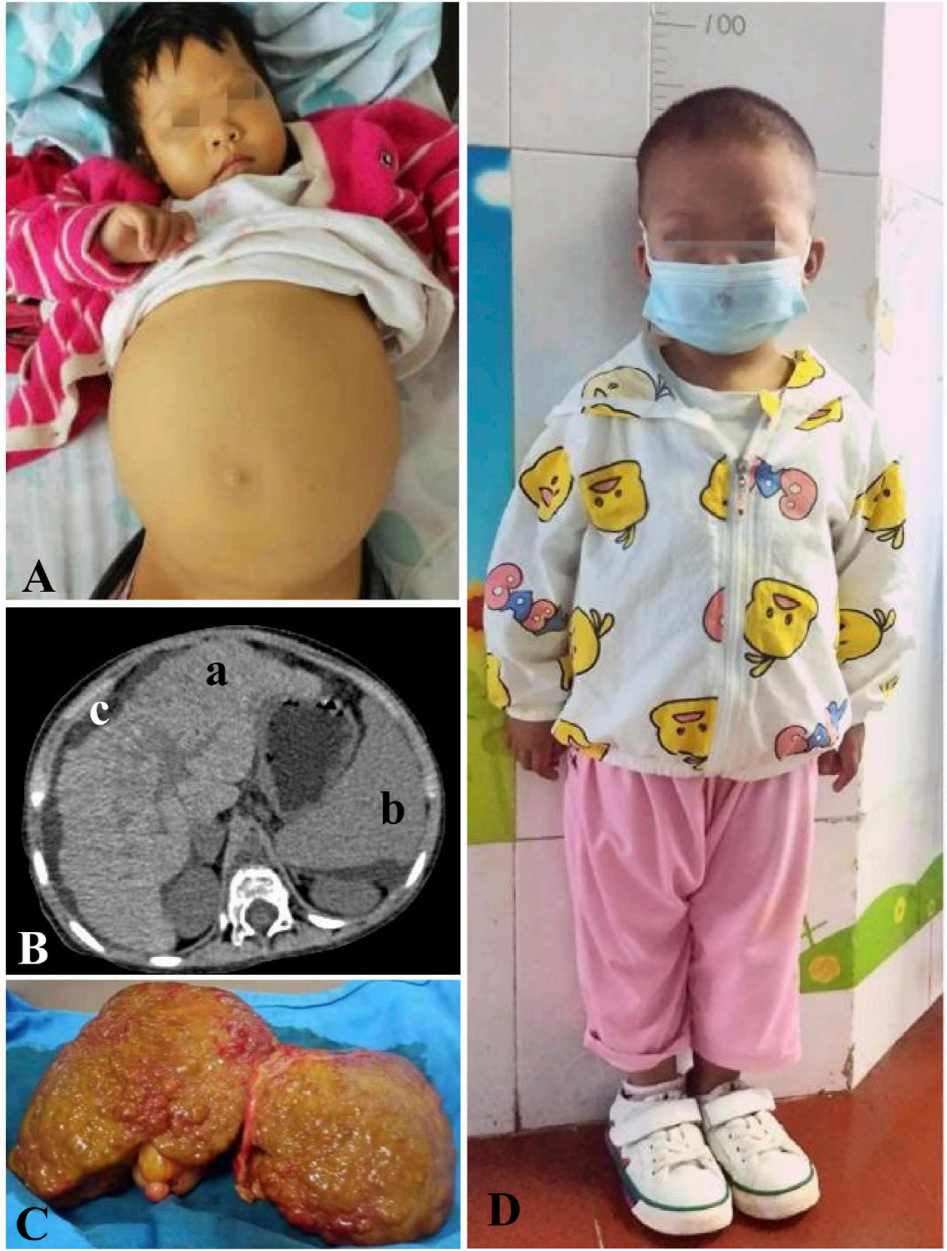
Clinical data of the proband. (A) End-stage liver disease, accompanying distension over the whole abdomen and severe yellowing of the skin. (B) Upper abdominal plain CT scan: (a). enlarged liver with a coarse, nodular texture and serrated capsule. (b). Enlarged spleen. (c). Ascites. (C) Lesion liver, an enlarged liver with a diffuse, gray–green coarsely granular appearance to the surface. (D) Proband 6 months after the transplantation, aged 3 years and 7 months, showing a symmetrical short stature with height 93 cm (3^rd^–15th) and weight 13.8 kg (15^th^–50th).
